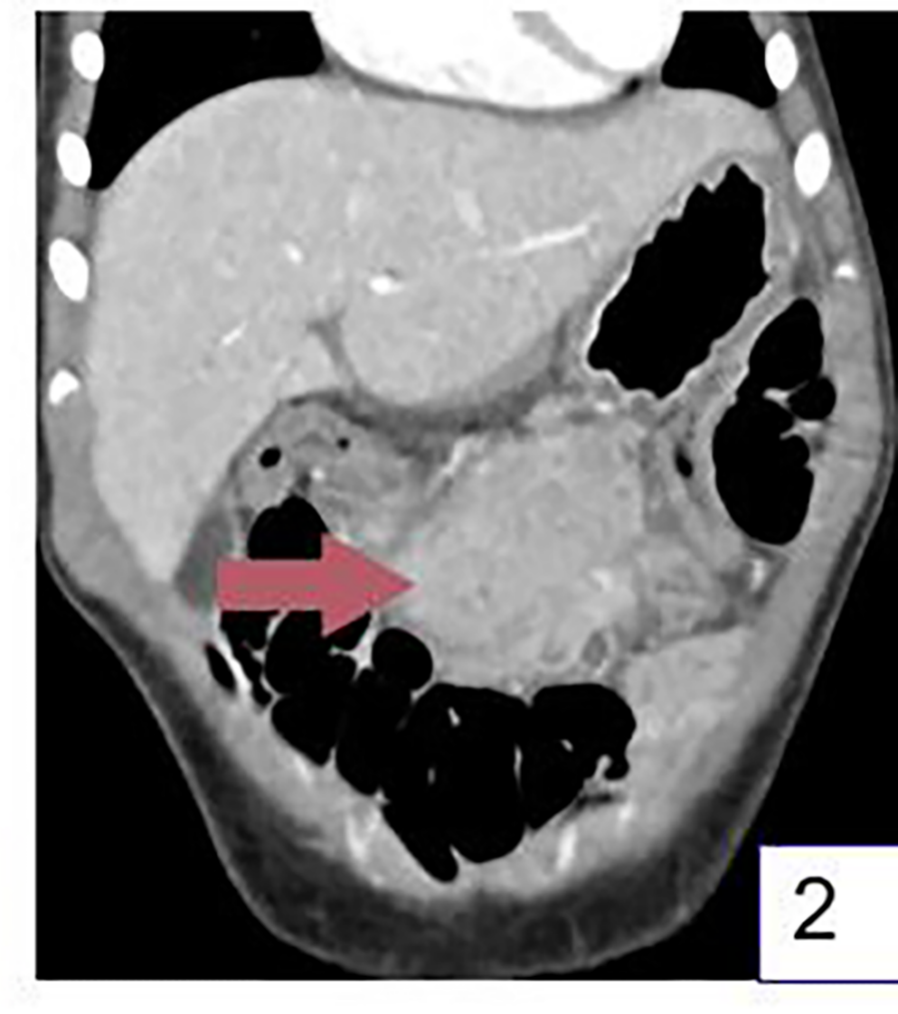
Coronal CT images showed the mass located between the stomach and colon. (Arrow shows the mass).
